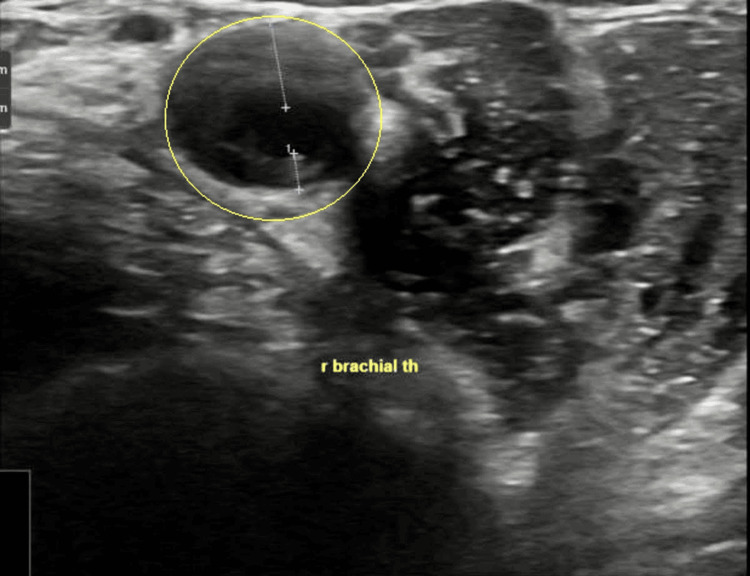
Ultrasound Doppler of the brachial artery Yellow circle points to the brachial artery halo sign
